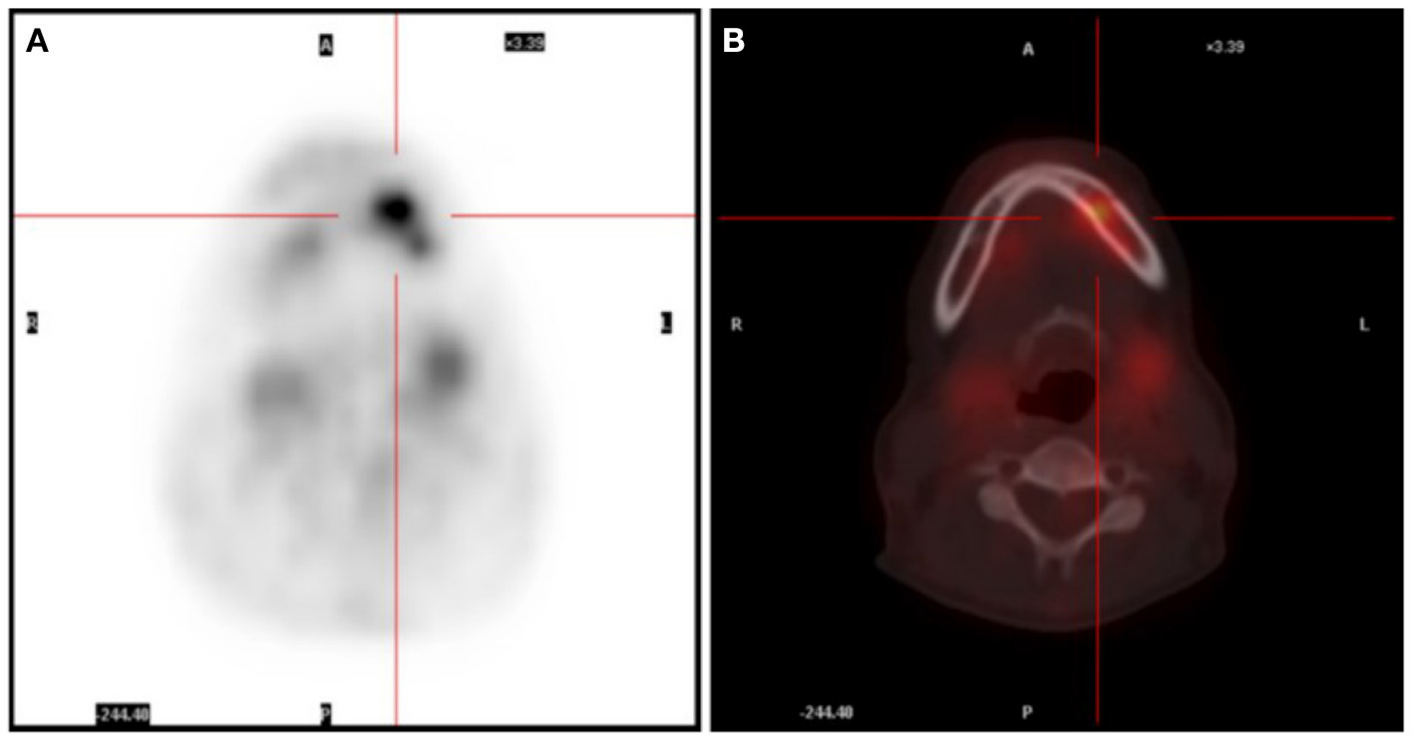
(A) PET scan showing an area with glucose uptake in the facial region. (B) PET/CT scan revealing the uptake area located in the left mandible.
